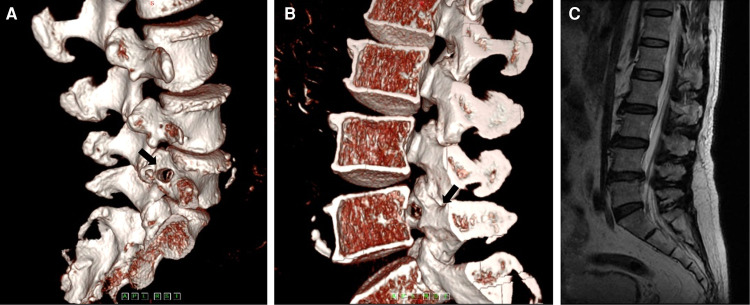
(A) The postoperative computed tomography shows the entrance of the bony channel on the pedicle. (B) The postoperative computed tomography shows the exit of the bony channel on the pedicle. (C) The postoperative magnetic resonance imaging shows no residual matter.
